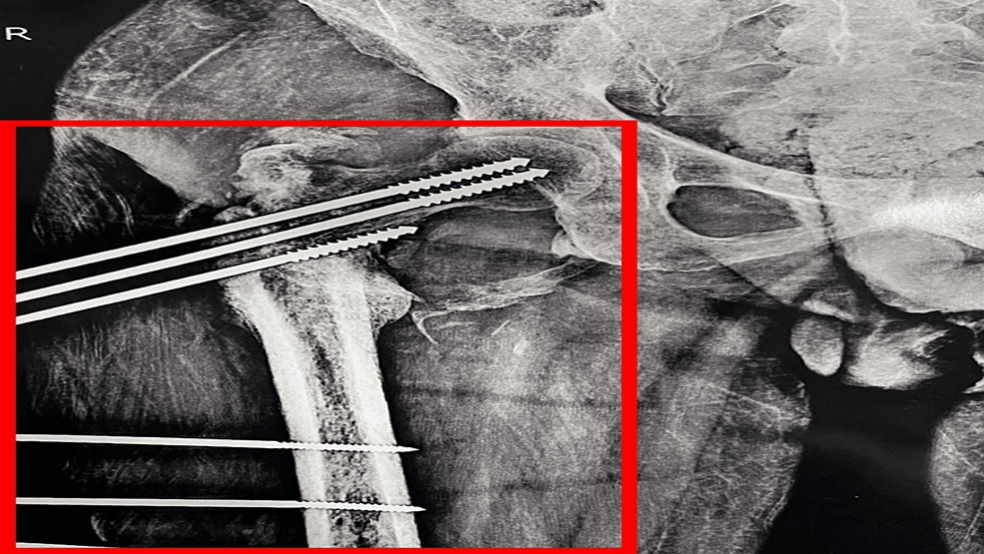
Postoperative X-ray image The image is showing an intertrochanteric fracture fixed with simple external fixation
